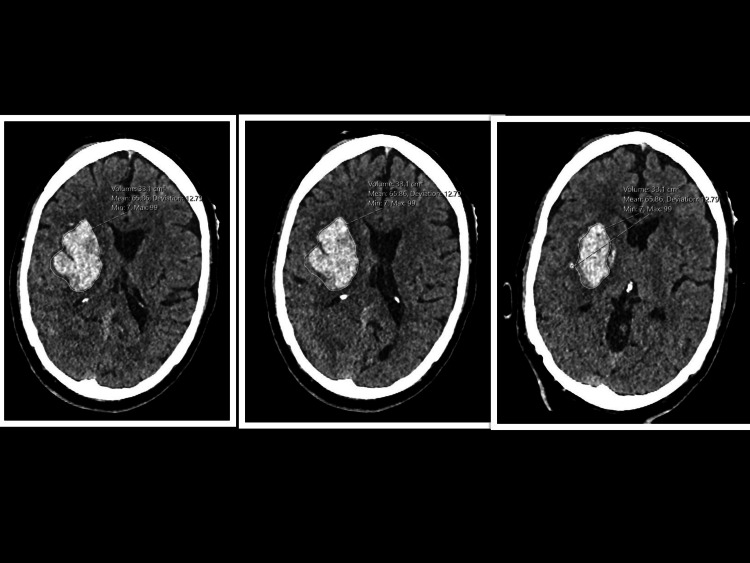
Non-contrast axial CT scan of the brain on presentation (Day 1). An acute right thalamoganglionic hemorrhage measuring approximately 33.1 cm³, with partial effacement of the overlying convexity sulci and adjacent ventricle, and a 5-6 mm leftward midline shift.
